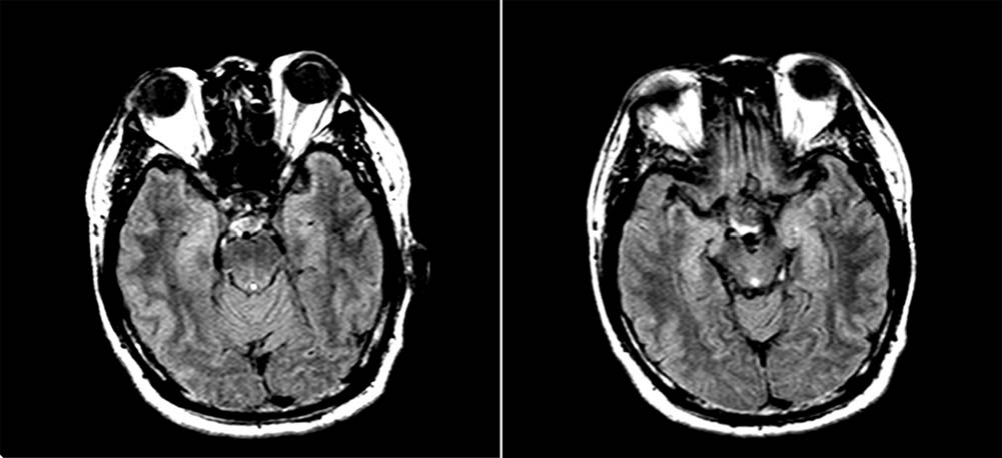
MRI T2/FLAIR shows hippocampal focal T2 hyperintensities with shrinkage in lateral ventricular temporal horn.
